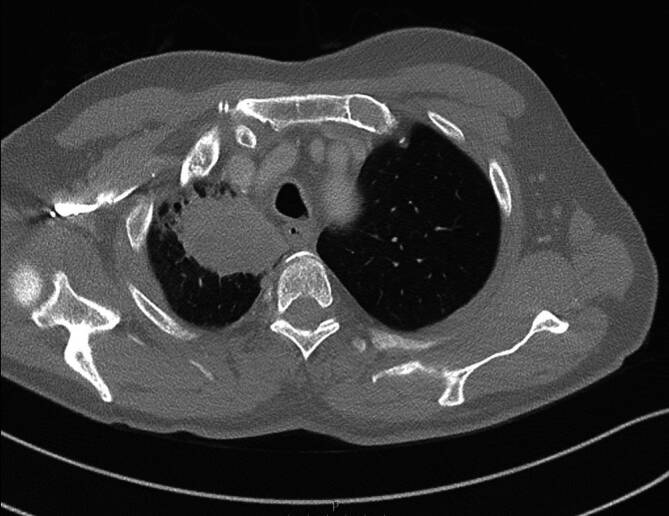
Transversale CT Thorax mit apikaler pulmonaler Raumforderung
